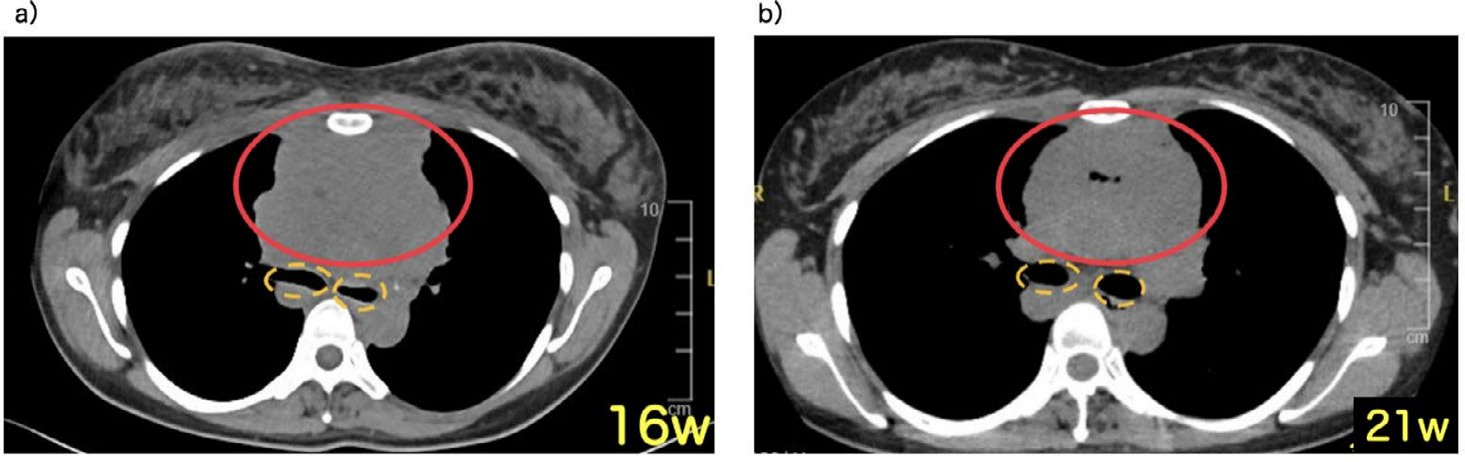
Chest computed tomography. (a) At 16 weeks of gestation (onset), a large anterior mediastinal mass (solid line) and compressed bronchus (dotted line) were observed, accompanied by superior vena cava syndrome and upper body edema. (b) At 21 weeks of gestation (after initiation of chemotherapy), the mediastinal mass (solid line) showed slight reduction in size, and the compressed bronchus (dotted line) was relieved. Upper body edema was also improved. Previously unseen intratumoral air accumulation was observed.
